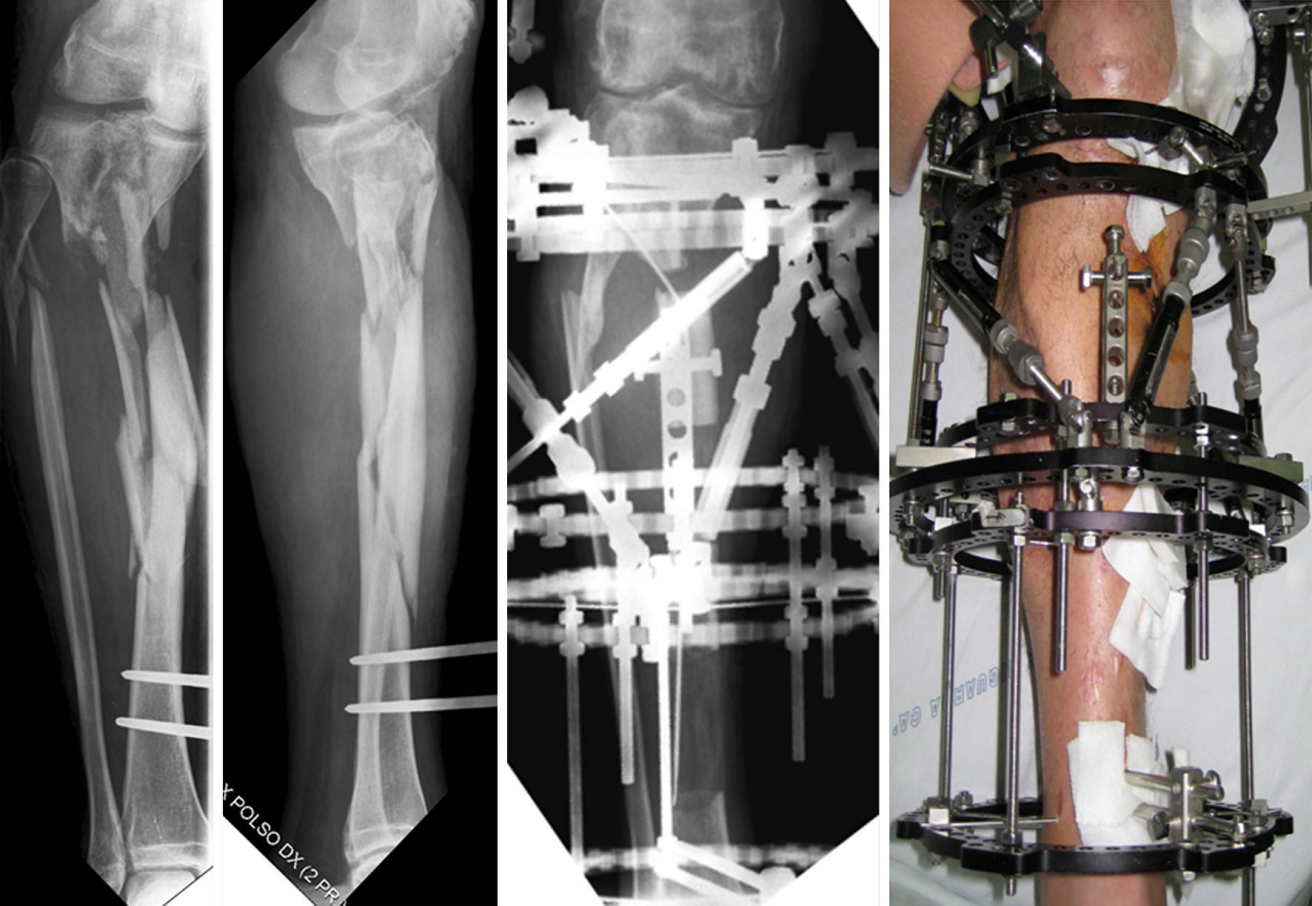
43-year-old man with infected tibial nonunion treated with bony resection of all infected bone and a trifocal retrograde tibial bone transport. From *left to right*, images show radiographs of the tibial nonunion with a temporary external fixator, anteroposterior radiograph with the TSF applied, and clinical photograph after application of the TSF during tibial bone transport
